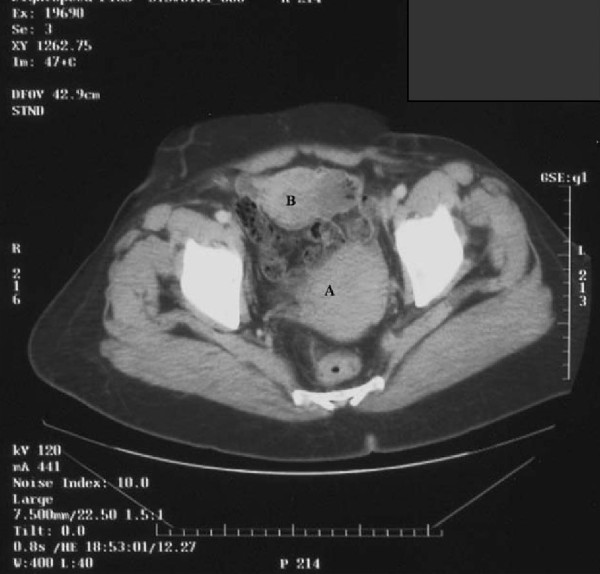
A computed tomography scan demonstrating a fibroid uterus (A), anterior to the uterus is an 8x 6x 6 cm mass (B).
